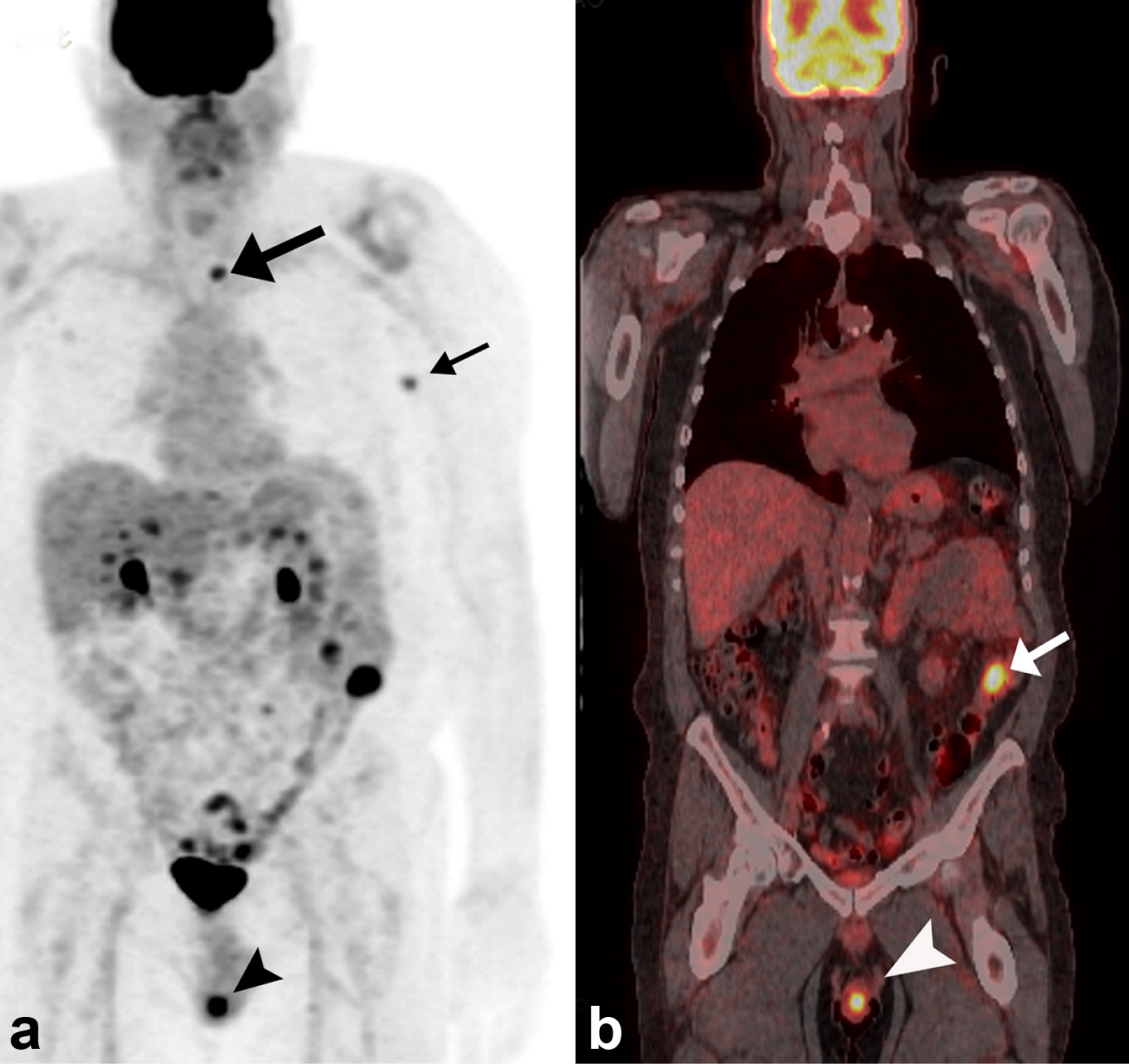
Positron emission tomographyPET-CT examination 8 months prior to presentation
